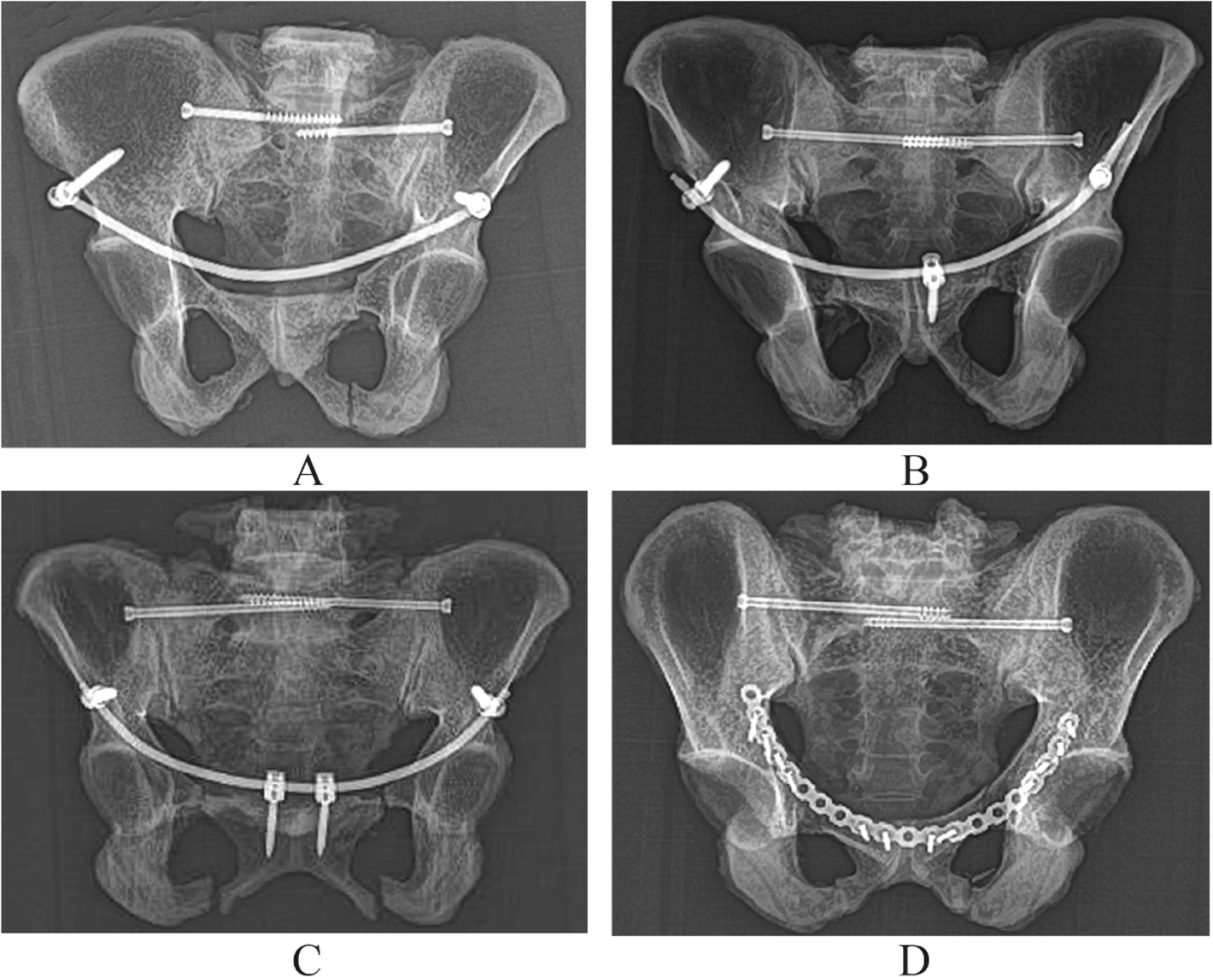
CT images of fixation models. a Fixation model 1. b Fixation model 2. c Fixation model 3. d Fixation model 4
